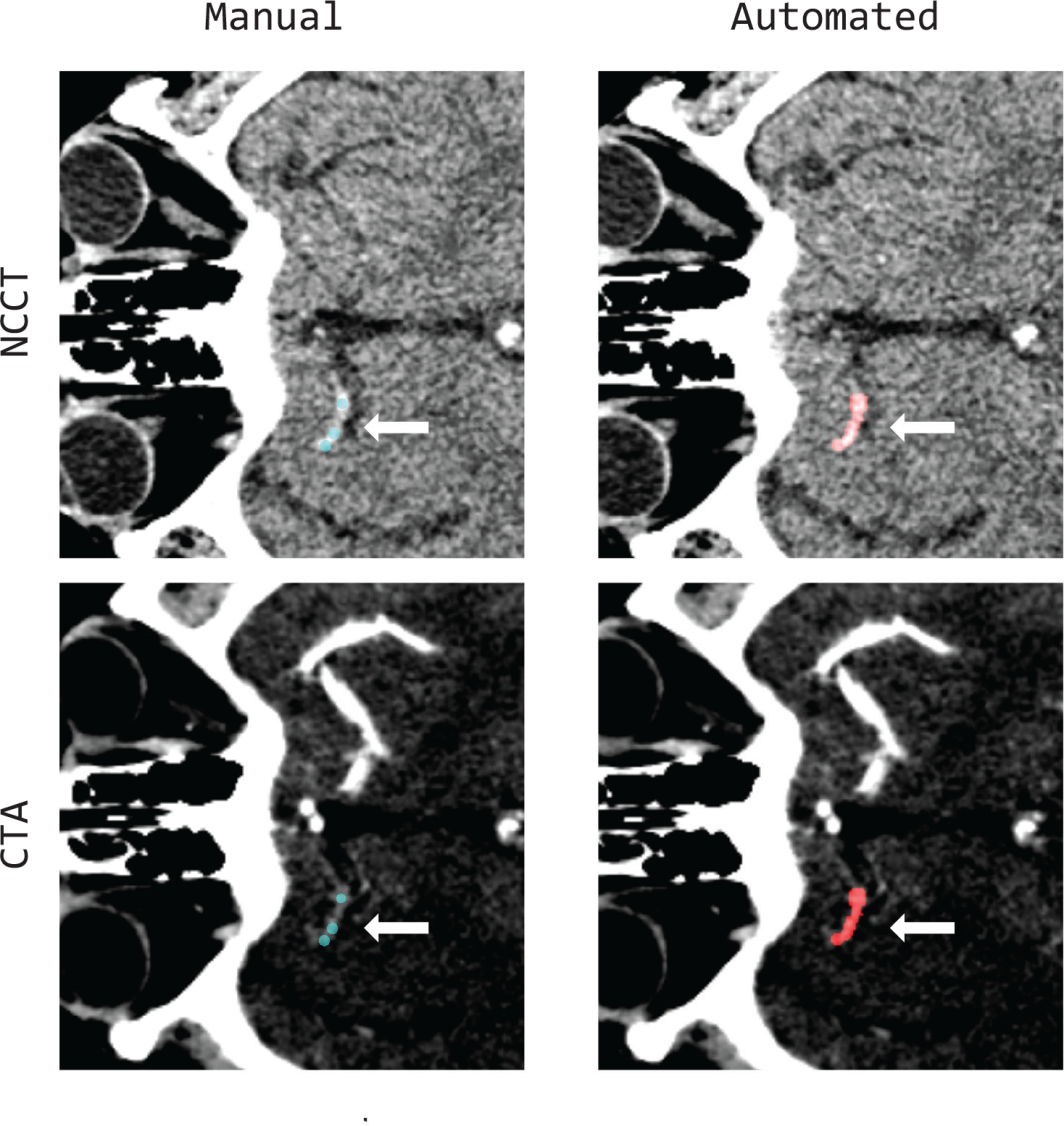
Example of registered NCCT (left column) and CTA (right column) images of a patient suffering from an M1 occlusion (white arrows). The green overlay (on the left) represents the manually measured density using the three spherical ROI. On the right panel, the red overlay highlights the result of the automated thrombus segmentation on CTA.
